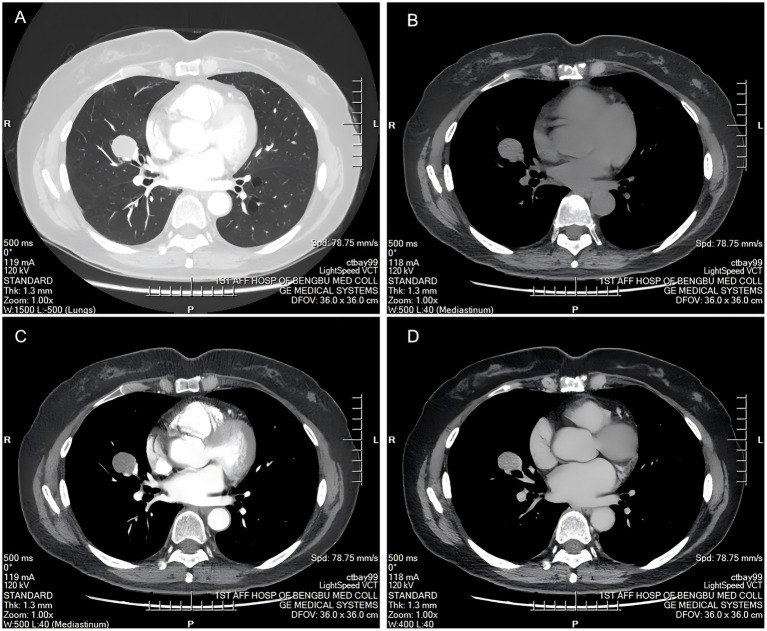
Typical solid nodule of pulmonary sclerosing pneumocytoma (PSP) on CT. White arrows indicate the lesion and marginal vessel sign. A well-circumscribed round solid nodule is visible in the medial segment of the right middle lobe. (A) Non-contrast lung window. (B) Non-contrast mediastinal window. (C) Arterial phase. (D) Venous phase showing progressive homogeneous enhancement.
